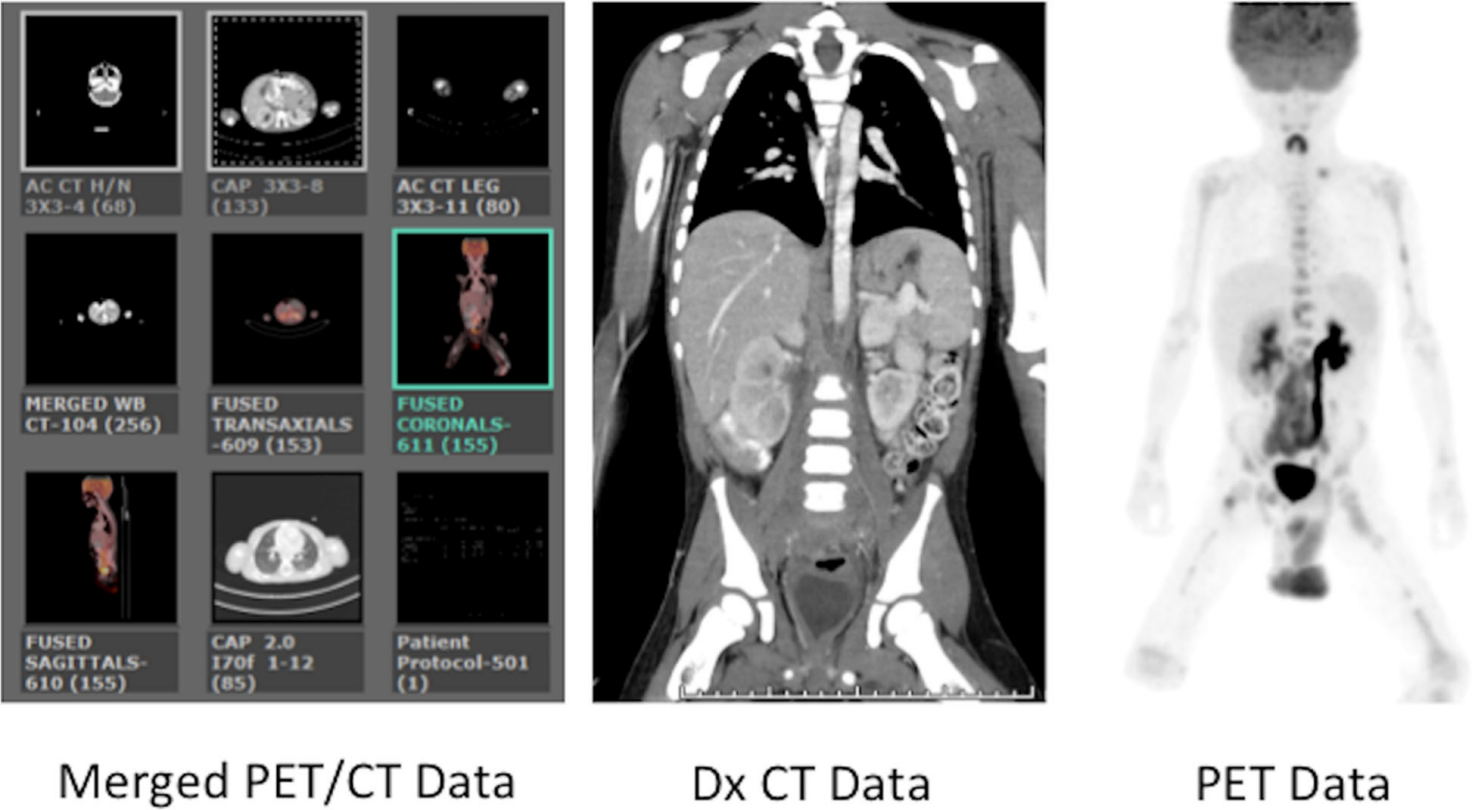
Multi-series PET/CT. Screen capture from the acquisition workstation shows the components of the multi-series PET/CT exam, in this case including low dose AC CT coverage of the Head/Neck, Dx CT coverage of the Chest, Abdomen, and Pelvis, and completion low dose AC CT coverage of the upper legs to match the PET acquisition. The merged CT data used to attenuation correct the PET data is displayed as a separate series at the workstation. Images from both the PET/CT and the diagnostic CT examinations are sent to their respective PACS exam folders and reported separately
